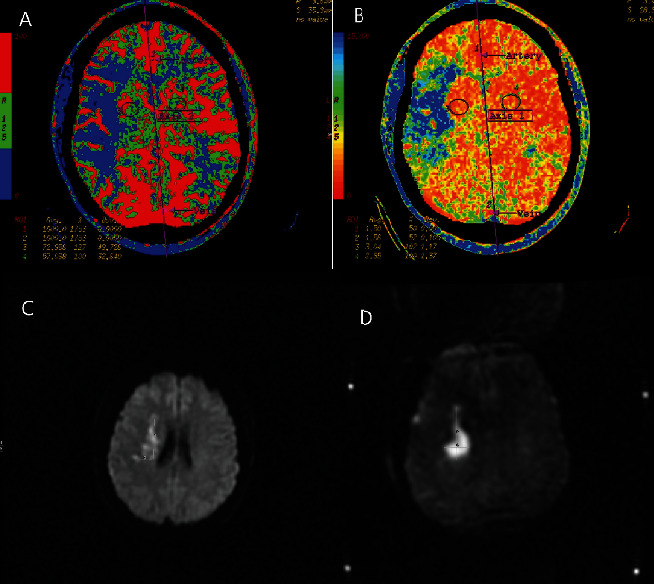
(a–d) CTA perfusion was obtained approximately 14 hours following presentation, following delivery by cesarean section. Perfusion maps demonstrate reduced blood flow (a) and reduced mean transit time (b) in the right MCA territory. Initial MRI (c) obtained within 3 hours following presentation demonstrates a focus of diffusion restriction in the right basal ganglia and corona radiata, measuring up to 4 cm compatible with core ischemic infarct. Postprocedure MRI (d), obtained after attempted thrombectomy and surgical endarterectomy, approximately 48 hours from the prior MRI, demonstrates stable 4 cm core infarct with sparing of the majority of the at-risk territory.
